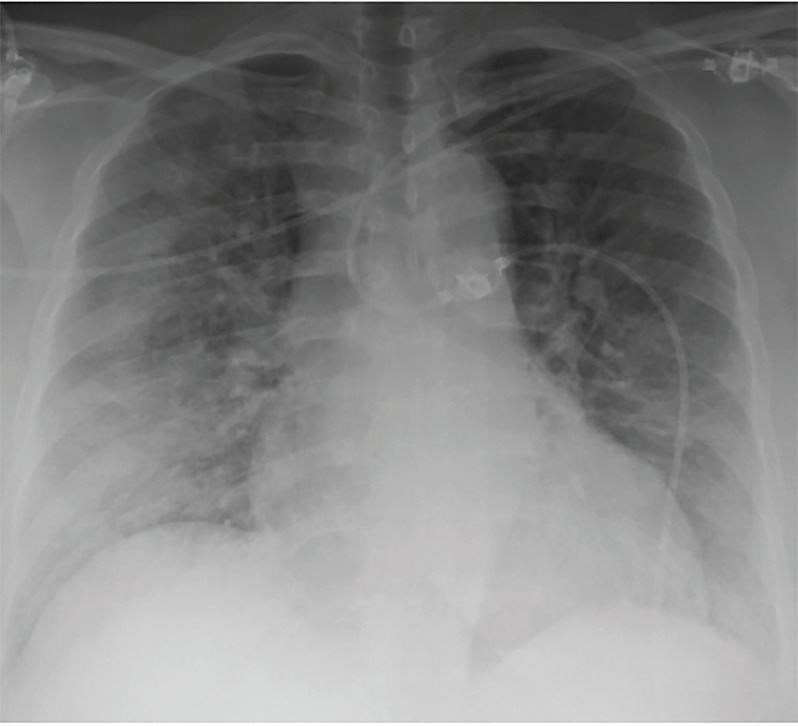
Anteroposterior chest radiograph in the emergency department showed bilateral pulmonary edema.
